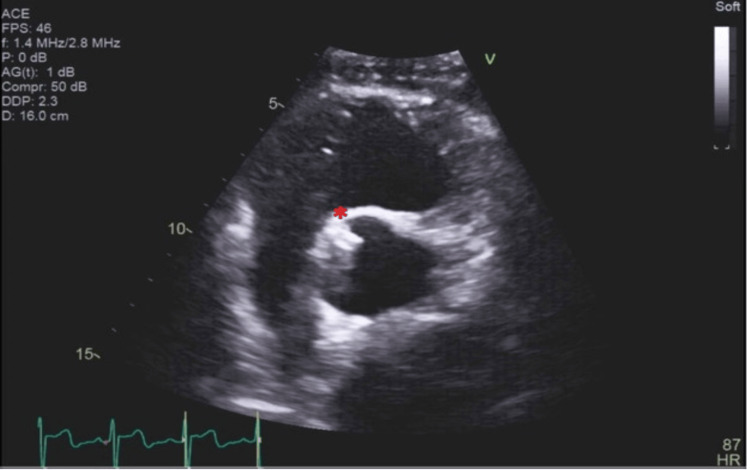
Transthoracic echocardiogram in a parasternal short-axis view Transthoracic echocardiogram (TTE) in parasternal short-axis (PSAX) view. Again, a tubular, echodense structure can be seen arising from the right coronary sinus, which protrudes into the aortic lumen at approximately the 11 o’clock position (marked with a red asterisk).
